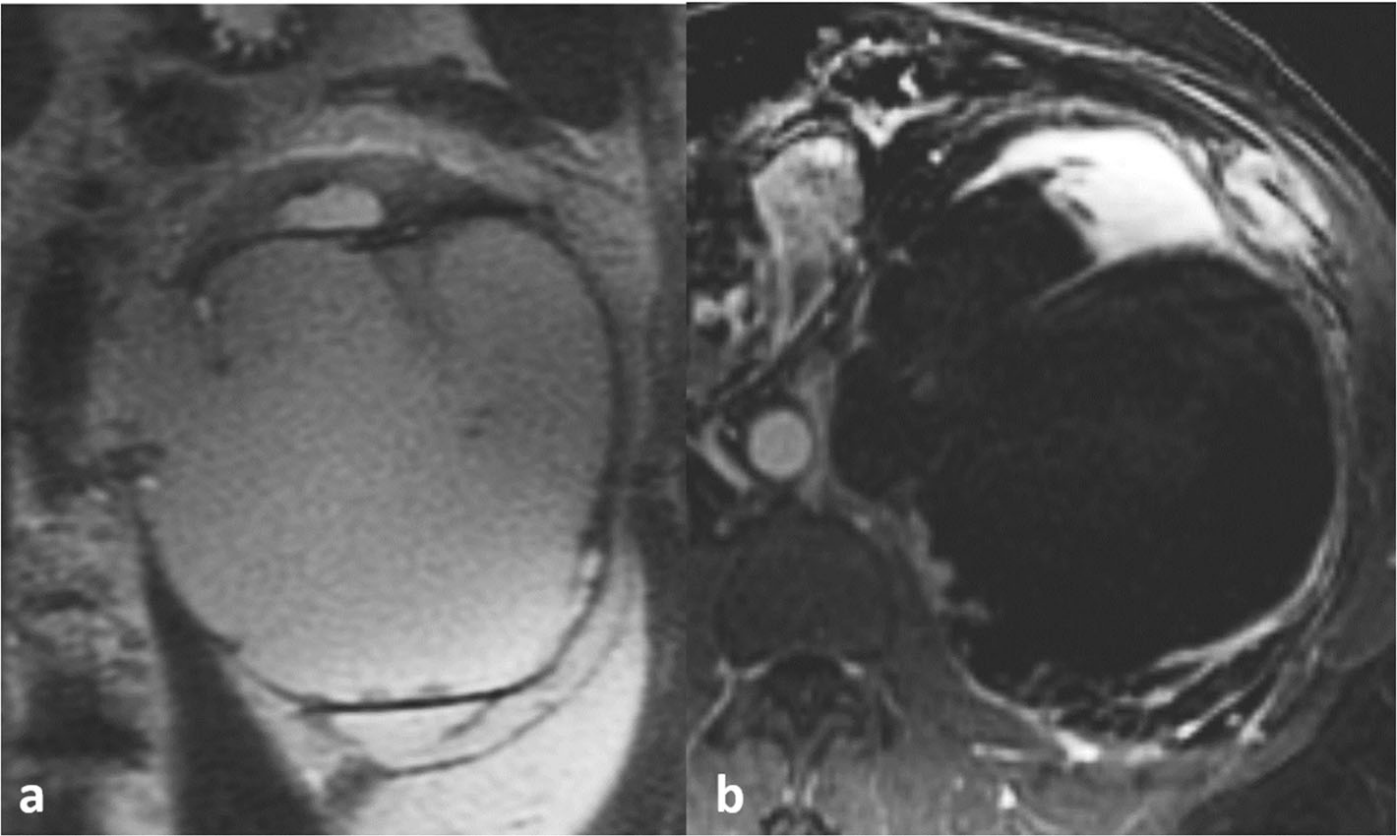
46-year-old female with fumarate hydratase-deficient renal cell carcinoma. On magnetic resonance imaging, axial T2 single-shot fast spin echo (a) and post-contrast 3D fat-saturated T1-weighted (b) sequences show a left renal mass with large cystic component
